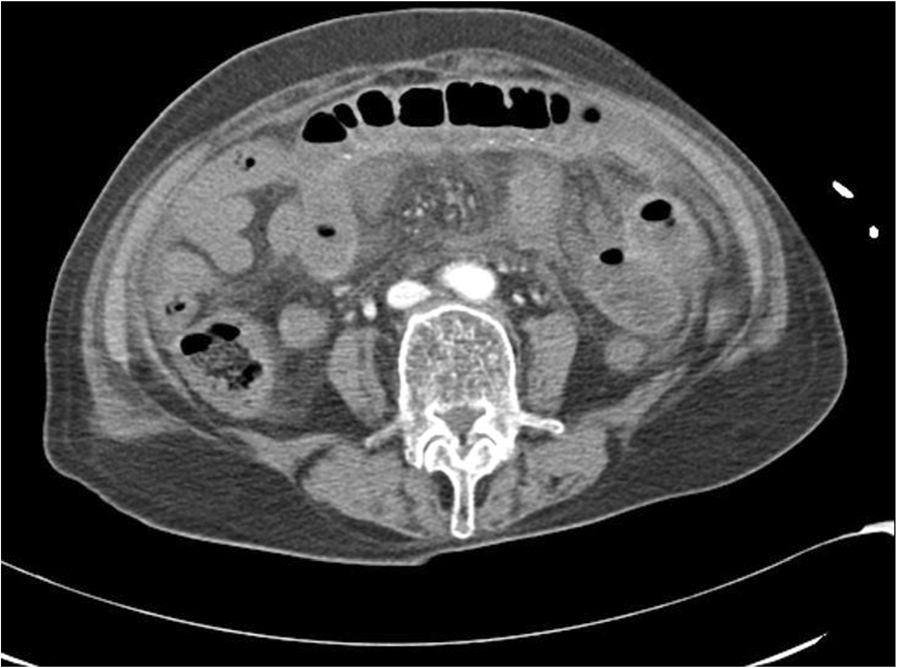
Computed tomography of abdomen shows intraperitoneal free air and decreased perfusion of the small intestine
